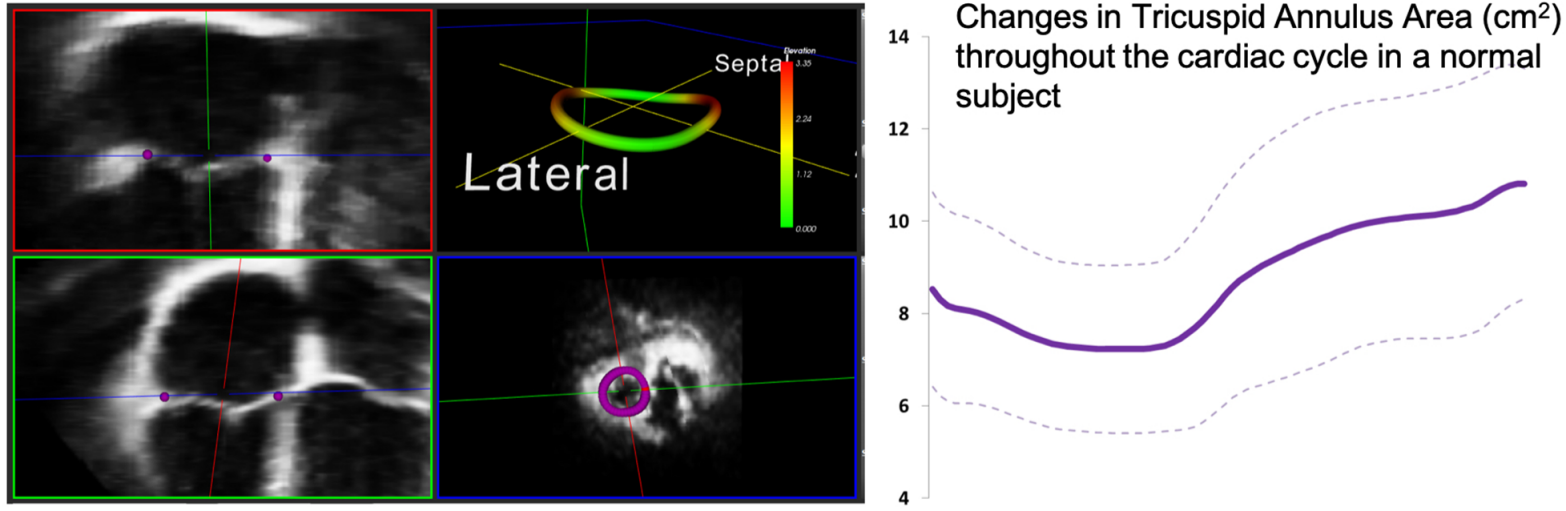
One example of customized software used to measure the 3D tricuspid annulus. Purple dots represent the initialization points from which the 3D annulus depiction (left panel, top right) is derived. The annulus is non-planar and therefore better assessed using 3D echocardiography. Dynamic analysis of the annulus allows calculation of changes in area and other parameters throughout the cardiac cycle (see graph right). Images courtesy of Federico Veronesi, PhD.
